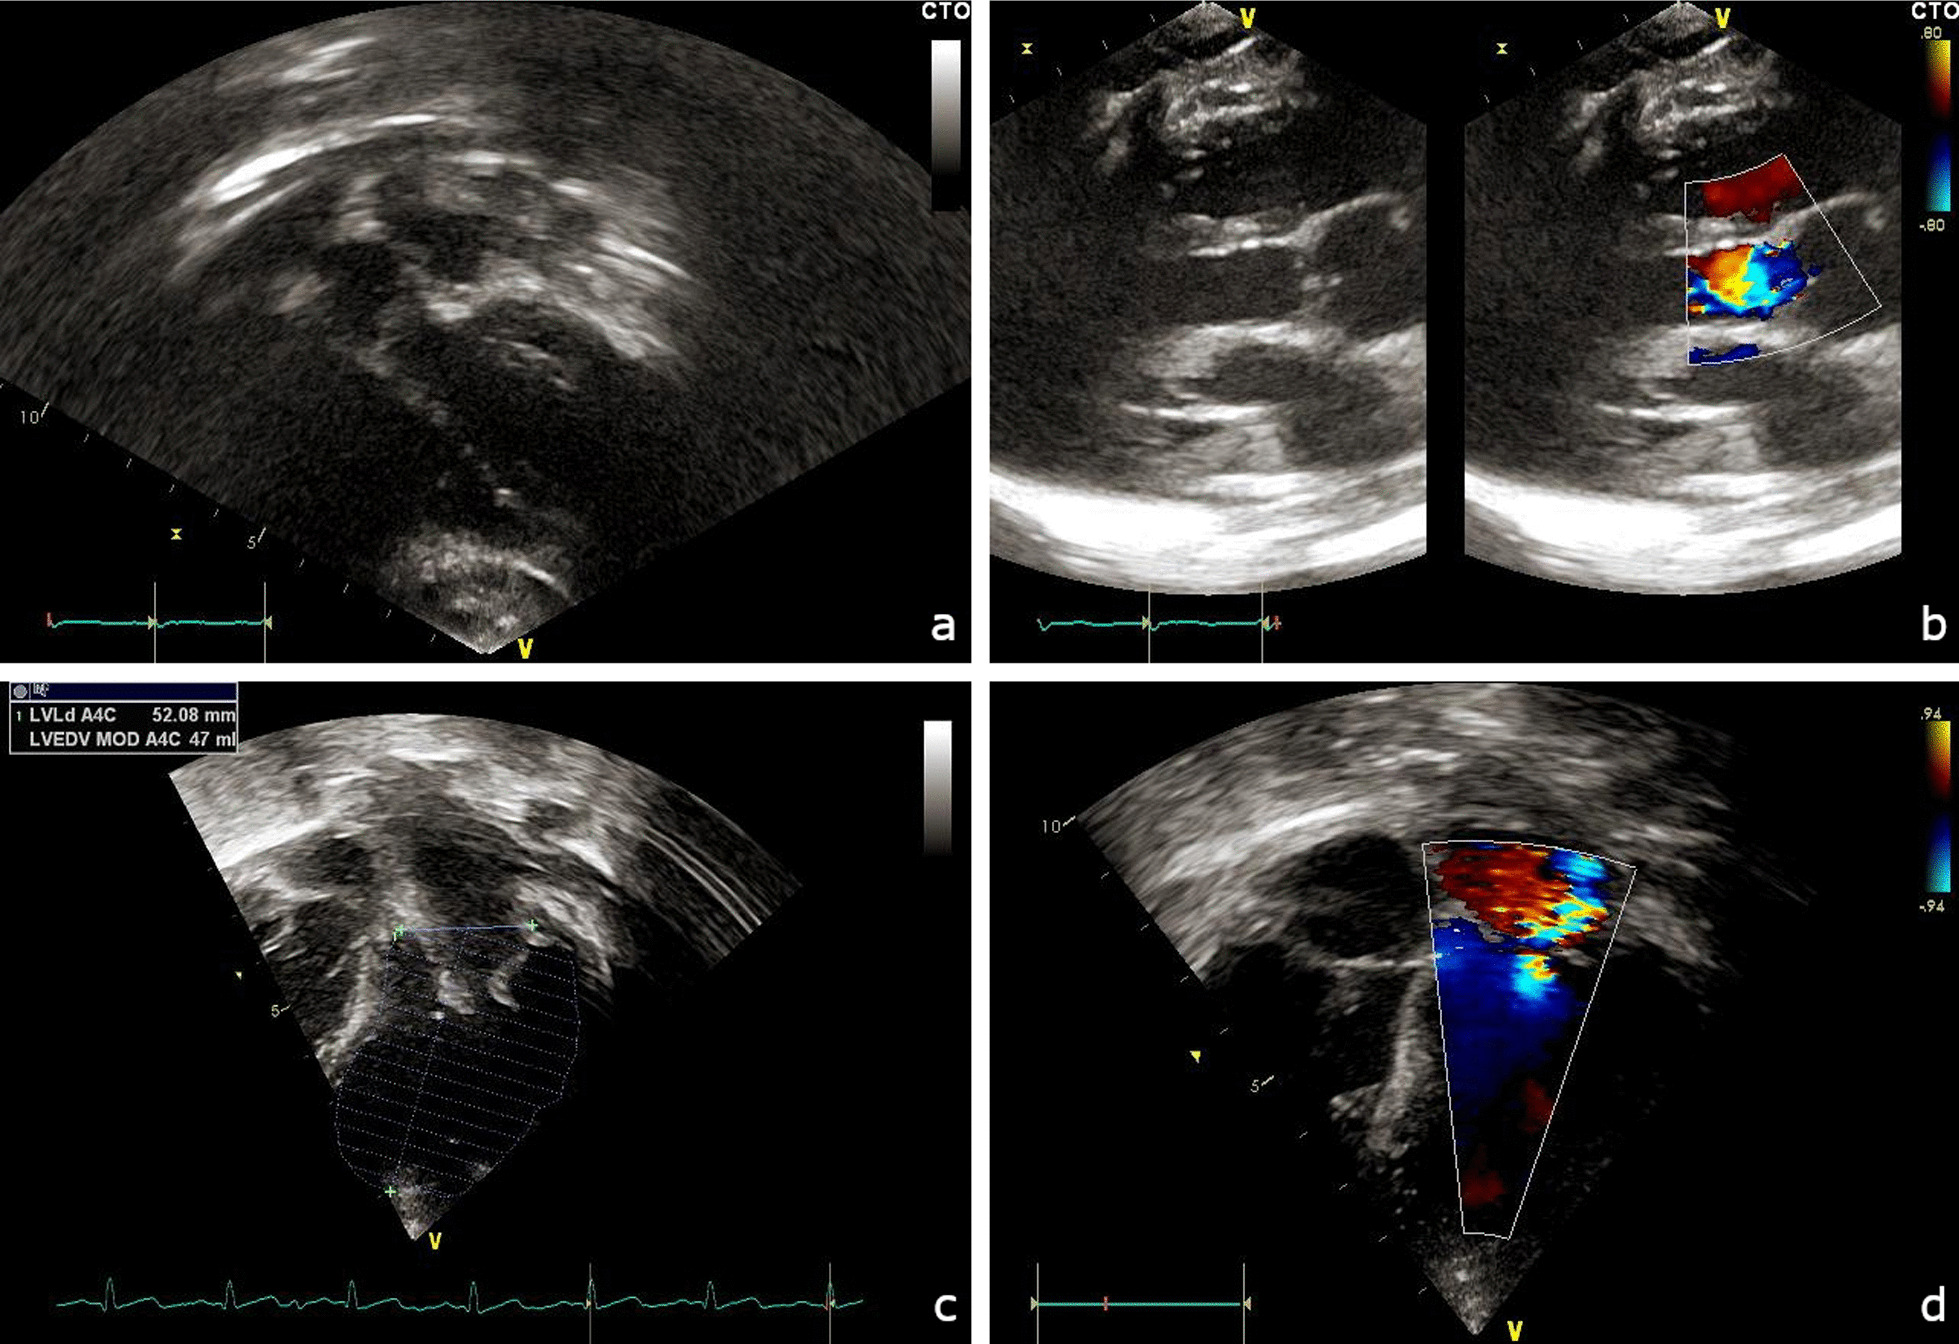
Representative echocardiographic images from two MPS VI patients. Aortic valve insufficiency in colour flow from apical four chambers view (a) and parasternal long axis (b), evidencing the thickness of valvular leaflets. Mitral valve in apical four chambers (c), showing mixomatous leaflets, and colour jet (d) evidencing a moderate-severe valve insufficiency
